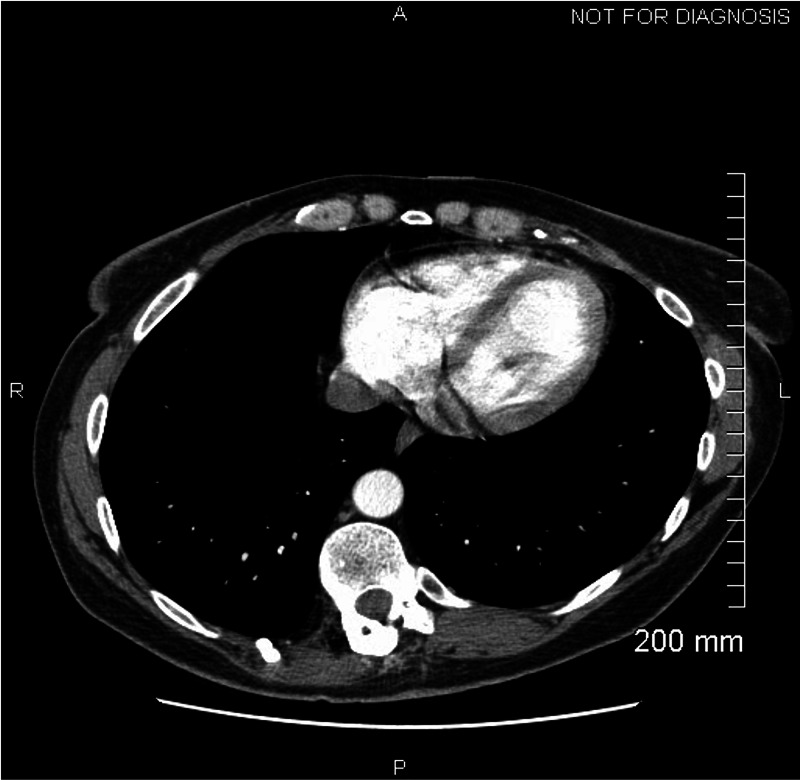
CT chest with contrast at a local hospital prior to transfer demonstrating no effusion.
